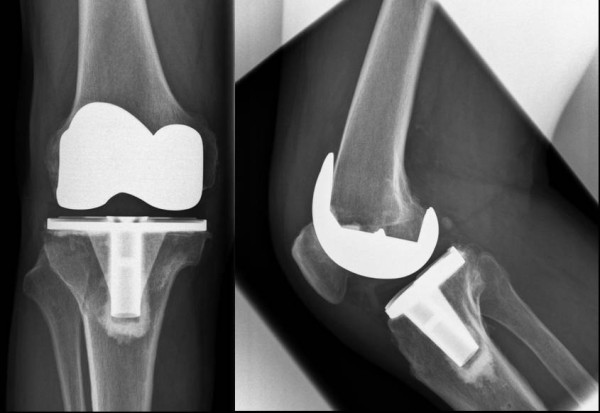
Implantation of a NexGen LPS TKA after previous closed-wedge osteotomy. The roentgenograms at the latest follow-up show good alignment and patella baja.
